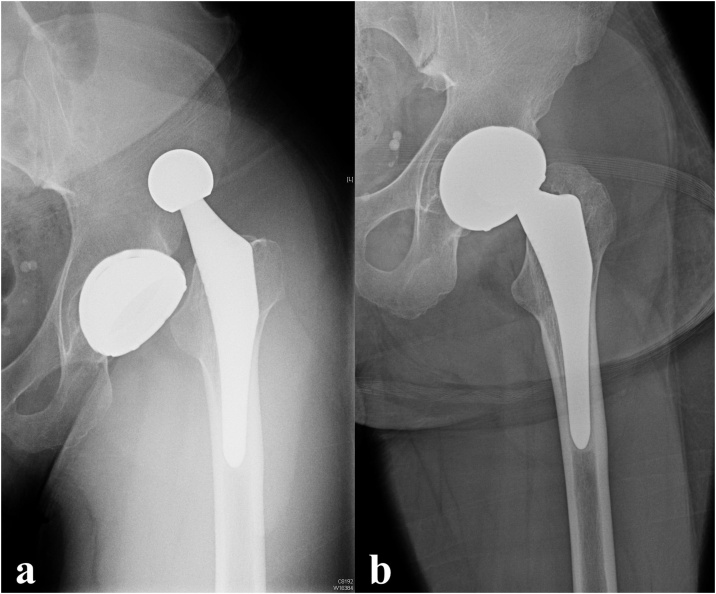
X-rays fromprevious recent hospitalization.
